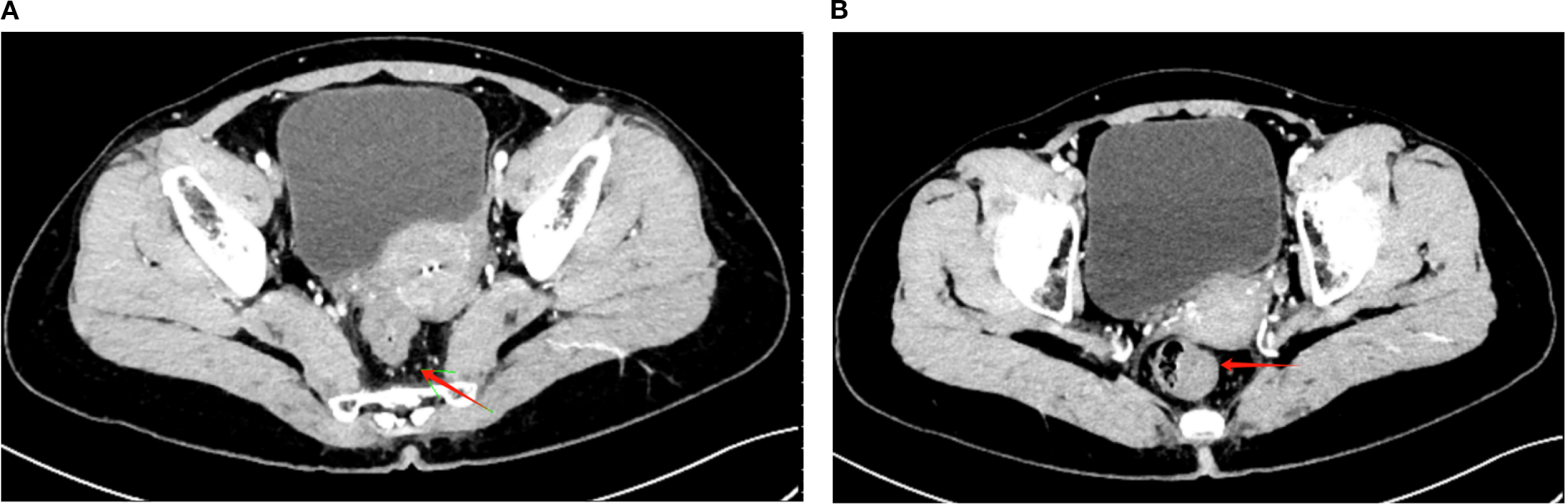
Post-treatment CT imaging showing tumor regression and lymph node reduction. (A) Axial CT image demonstrating a significant reduction in the rectal tumor volume (red arrow) following long-course radiotherapy (50 Gy/25 fractions) combined with concurrent chemotherapy (irinotecan + capecitabine). The serosal involvement is notably reduced, with clearer surrounding fat planes. (B) Axial CT image showing the reduced size of perirectal lymph nodes (red arrow), with partial regression of some nodes and no newly enlarged or suspicious lymph nodes observed.
